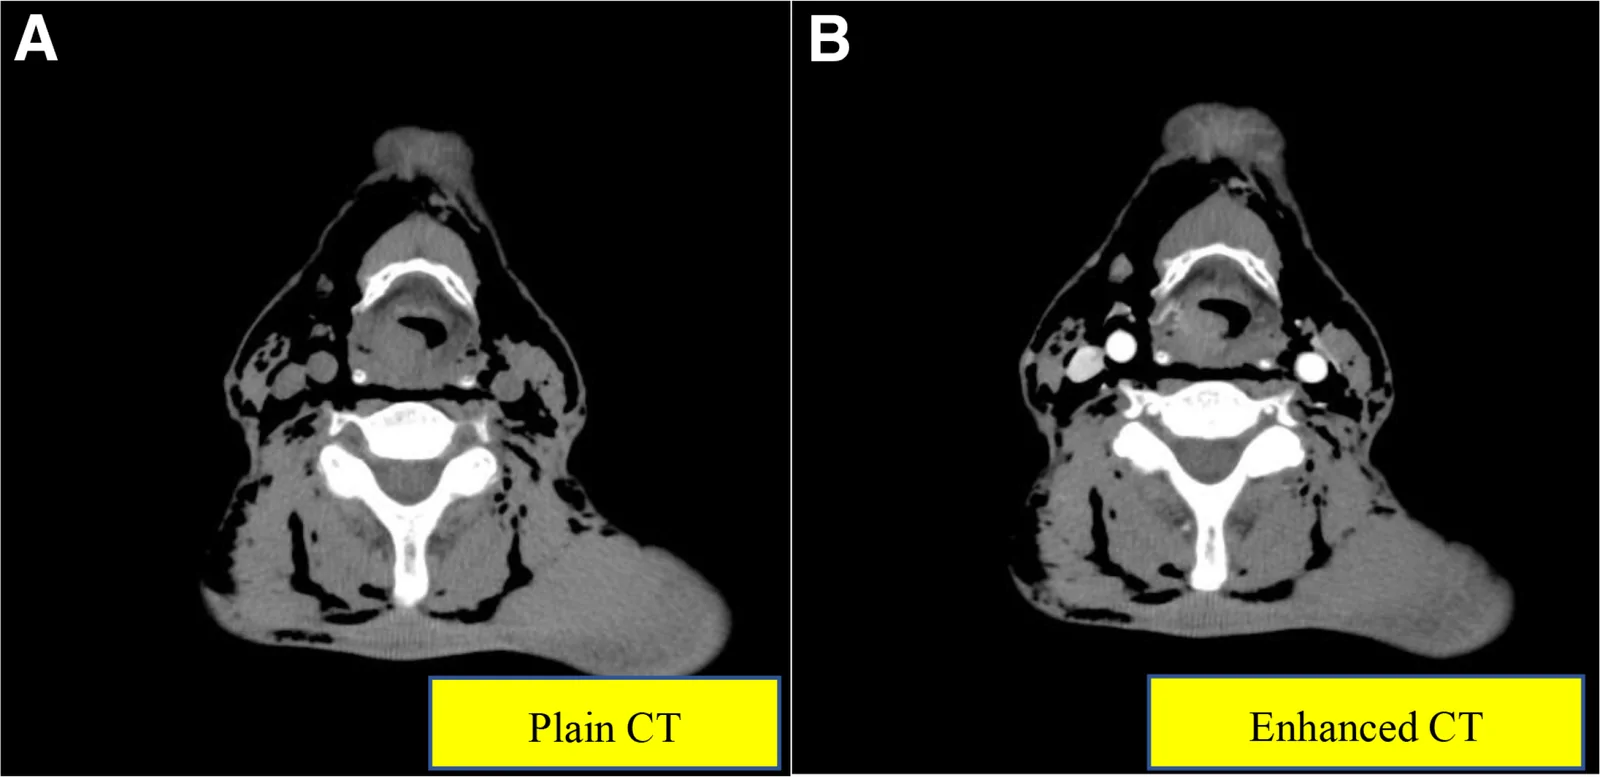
After biopsy, a plain CT scan of the larynx revealed a soft tissue density nodule in the right supraglottic area, with a maximum of 2.1 × 1.9 cm, poorly defined boundaries and uniform density, with an average CT value of about 55 Hu (A). Enhanced CT showed uniform enhancement of the lesions, with an average CT value of about 67 Hu, and thickened branch blood supply of the superior laryngeal artery (B). CT = computed tomography.
